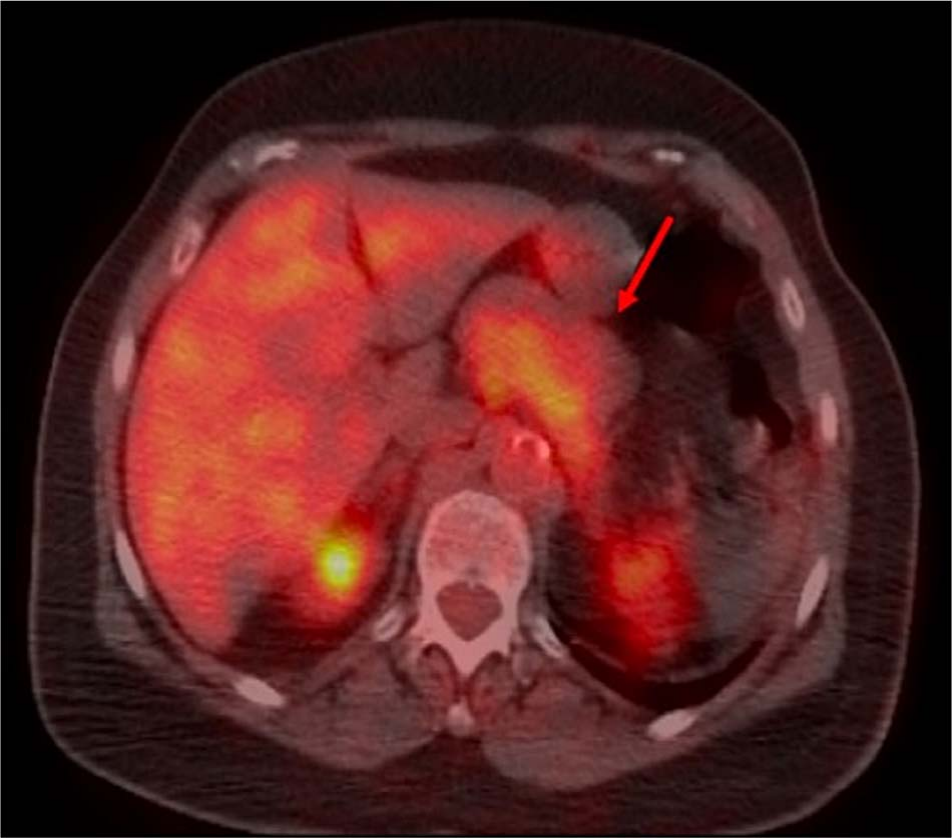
FDG PET scan demonstrating hypermetabolism of the mass.
